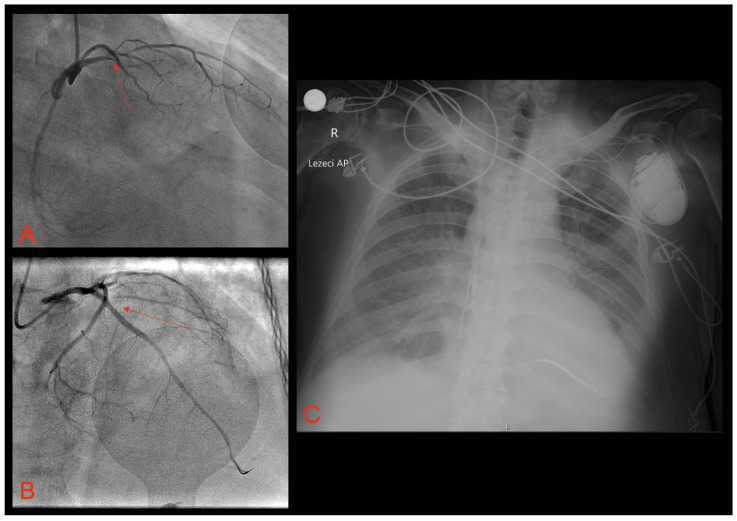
(A) Coronary angiography showing acute occlusion of the proximal left anterior descending artery (LAD); (B) coronary angiography showing established TIMI 3 flow through previously occluded LAD, following percutaneous coronary intervention and drug-eluting stent implantation; (C) antero-posterior chest X-ray showing the implanted ICD. Red arrows show a culprit lesion in the LAD.
